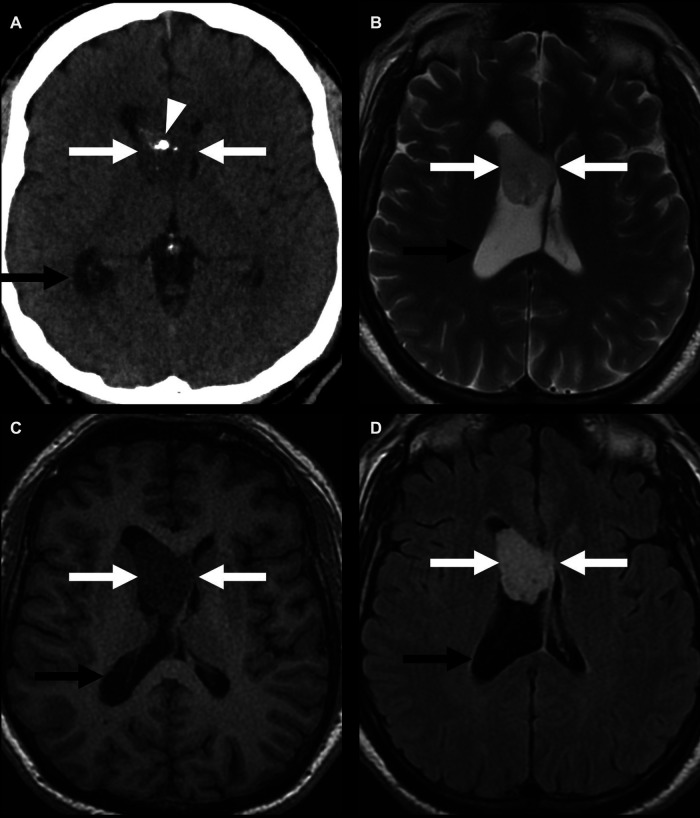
Lateral-ventricular subependymoma in a 63-year-old man (arrows). CT shows a mass in the frontal horn of the right lateral ventricle containing calcifications (arrowhead) (A). This mass is mildly T2 hyperintense (B) and T1 hypointense (C) and shows homogeneous post-contrast enhancement (D). The associated obstructive hydrocephalus of the right lateral ventricle (black arrow) is due to blockage of the foramen of Monro.
